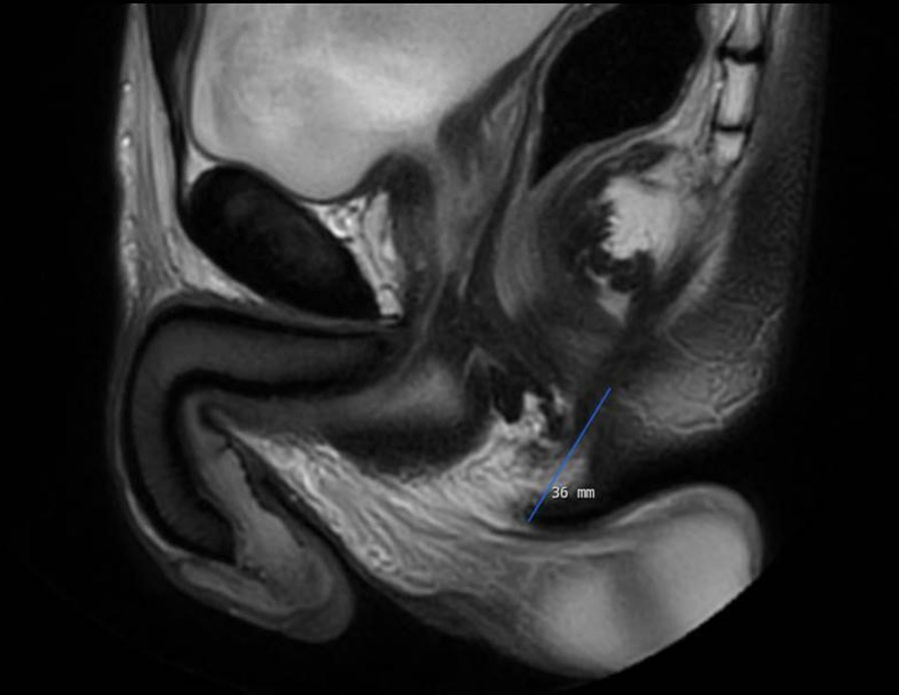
shows a sagittal MRI image of non-TML patient with AGD measurement of 36 mm. The measurement was performed using a straight line from the center of the anus to the posterior base of scrotum
